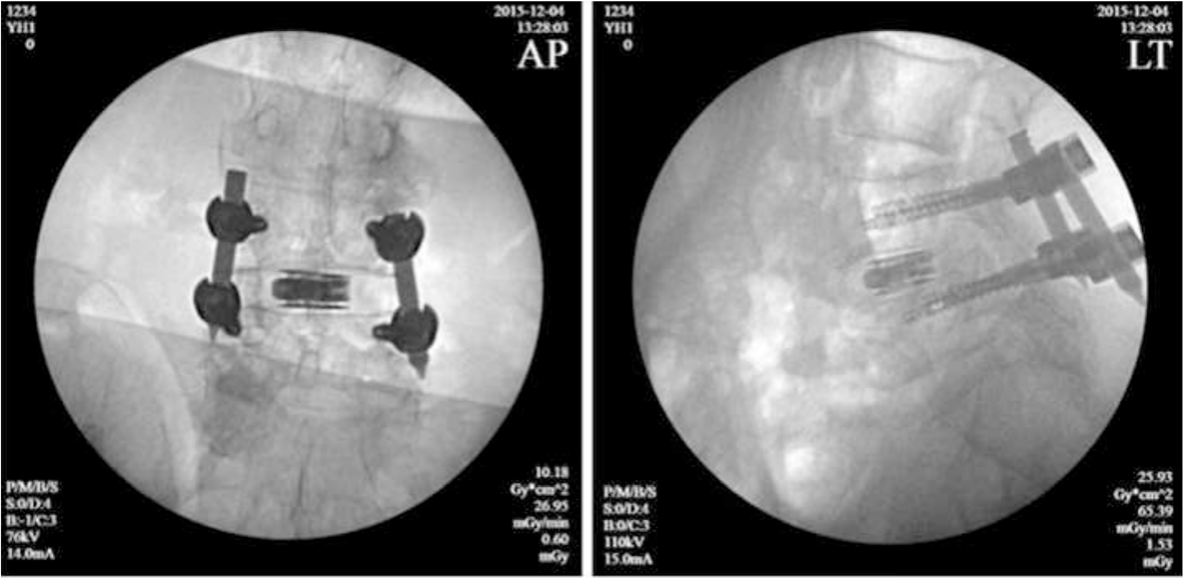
X-ray images showed the position of internal fixation and cage were favorable
